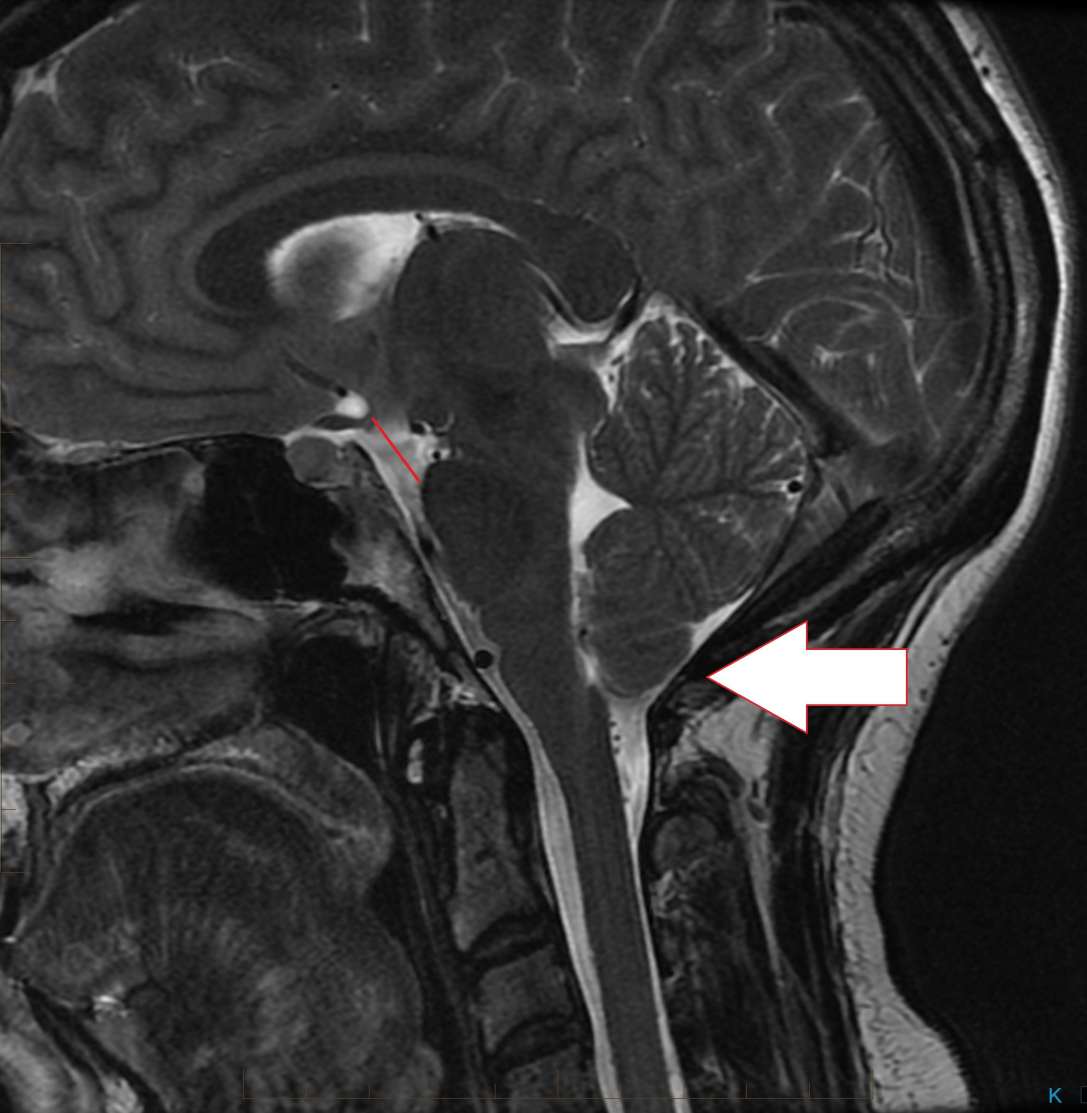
Sagittal T2 brain MRI demonstrating low-lying cerebellar tonsils with a decrease in the mamillo-pontine distance and draping of the optic chiasm over the dorsum sellae
